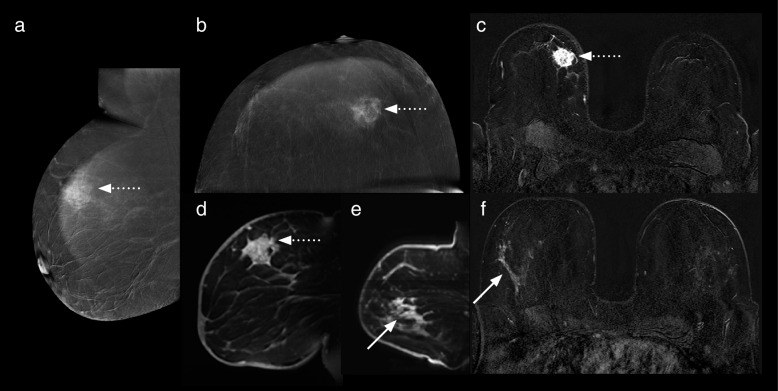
Case number 2 of Table 3. A 41-year-old woman with a palpable lump in the right breast. Recombined MLO (a) and CC (b) CEM images and first contrast-enhanced subtracted T1-weighted axial (c) and sagittal (d) MRI images. The index malignant lesion was a triple-negative, grade 3 invasive ductal carcinoma, seen here as a 15-mm ill-defined, irregularly shaped, heterogeneously enhancing mass (dotted arrows) at the 11 o’clock position in the right breast. Sagittal (e) and axial (f) post-contrast T1-weighted subtracted images from the MRI study demonstrate an additional lesion in the right lower outer quadrant, a 34-mm segmental area of non-mass enhancement (solid arrows), not visible on CEM. This additional lesion was 30-mm low-grade DCIS. The degree of enhancement of the index lesion on MRI is subjectively much greater than that shown on CEM. The noncalcified DCIS shows minimal enhancement on MRI. *CC* Craniocaudal, *CEM* Contrast-enhanced mammography, *DCIS* Ductal carcinoma *in situ*, *MLO* Mediolateral, *MRI* Magnetic resonance imaging
